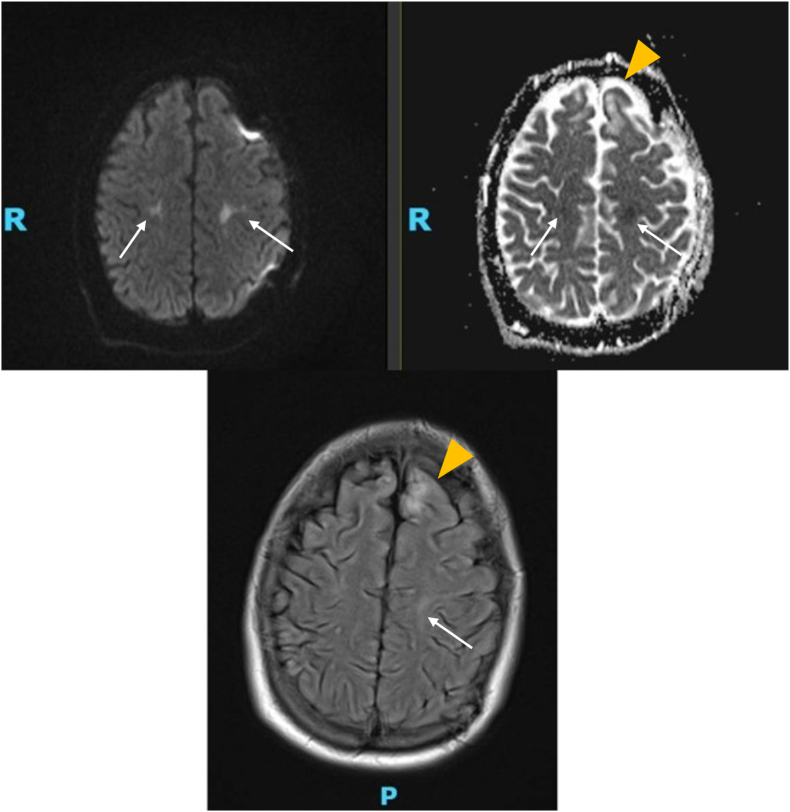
Left: DWI sequence. Right: ADC sequence. Bottom: T2-weighted FLAIR image. Encephalomalacia of the bilateral superior frontal gyri, larger on the left hemisphere compared to right, due to known history of traumatic brain injury. Moderate areas of subcortical or juxta-cortical white matter abnormalities high in the posterior frontal lobes bilaterally on DWI with associated ADC dropout representative of true diffusion restriction consistent with acute ischemic infarct. Images were obtained using a 1.5T MRI scanner. DWI acquisitions were trace-based with 3-mm slice thickness and 0.4-mm interslice gap. ADC sequences were acquired with identical parameters. T2-FLAIR images were acquired with a 5-mm slice thickness and 0.65-mm interslice gap.
